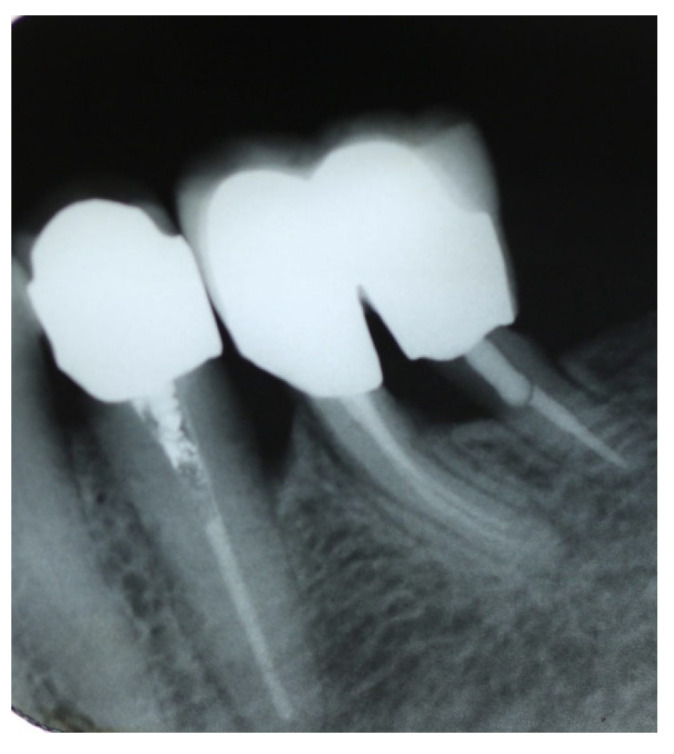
Recall X-ray after 6 months of prosthetic rehabilitation.
